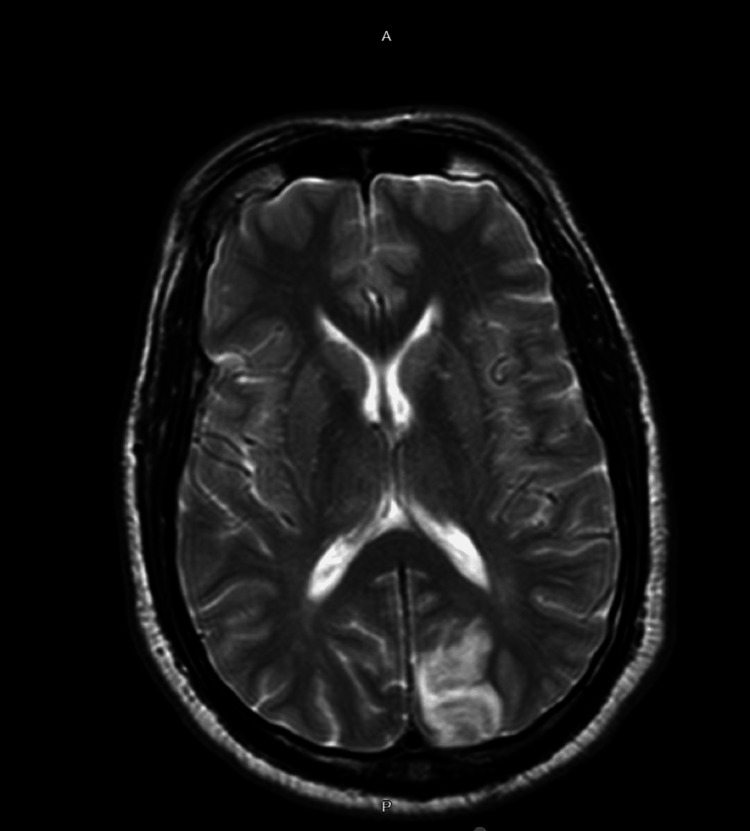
MRI brain T2W images showing left occipital lobe ischemia with a hemorrhagic component MRI: Magnetic resonance imaging; T2W: T2 weighted
